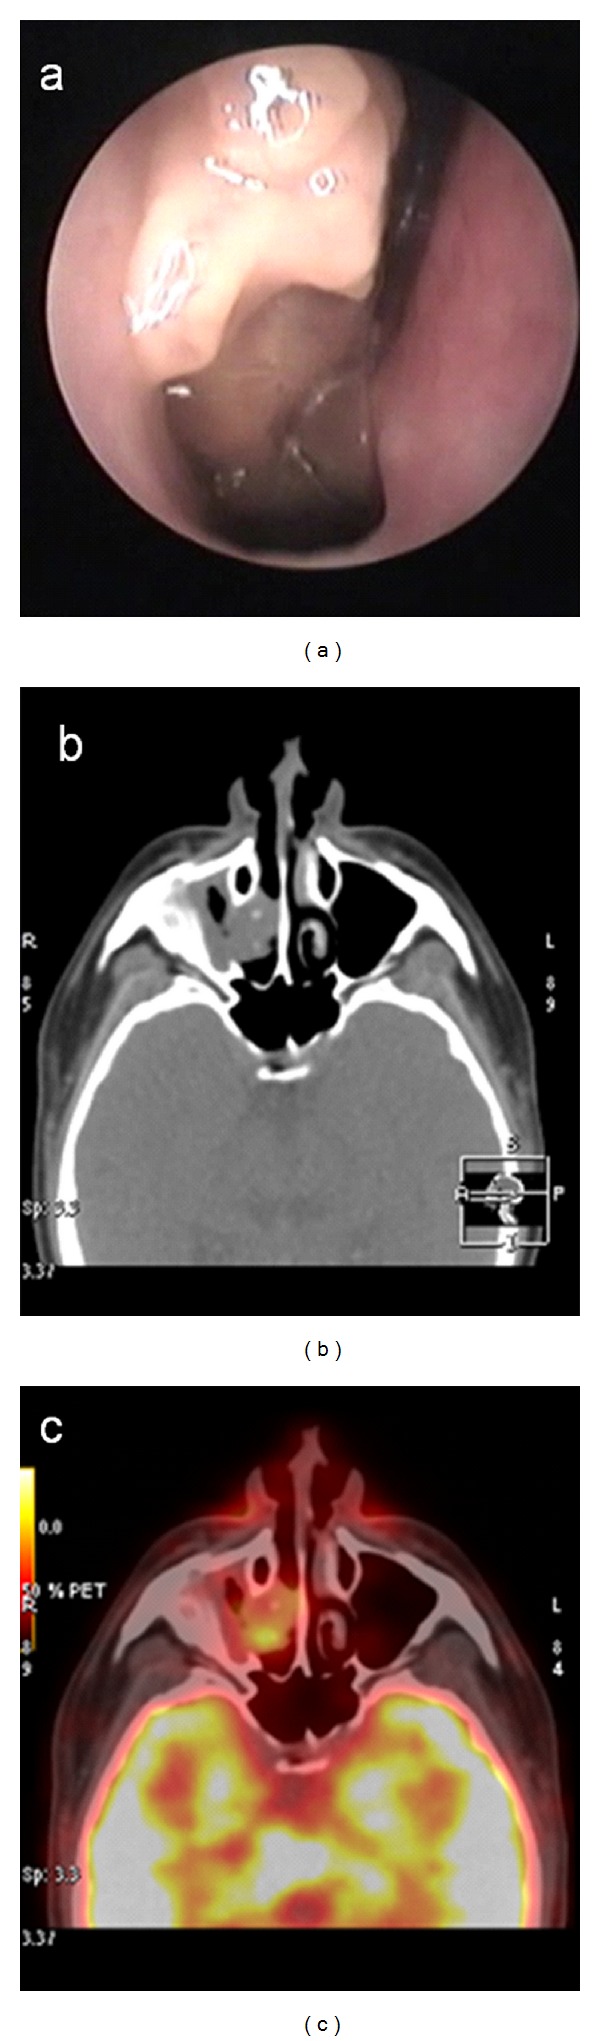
Case  11: (a) endoscopic view, (b) CT, and (c) ^18^FDG-PET/CT findings (SUV_max⁡_ 5.6).
